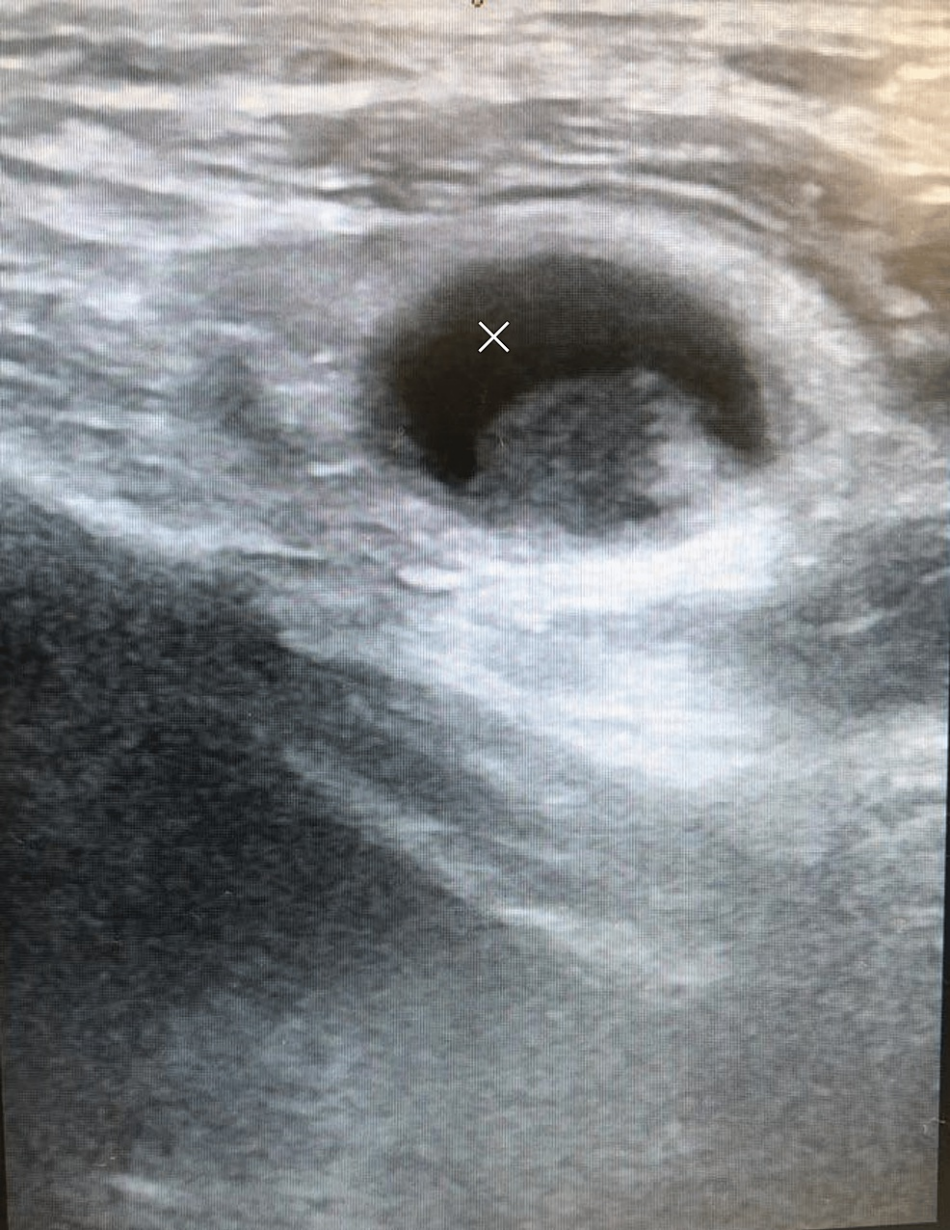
POCUS of the inguinal region showing the obturator hernia in the longitudinal plane (white star). POCUS: point-of-care ultrasound
